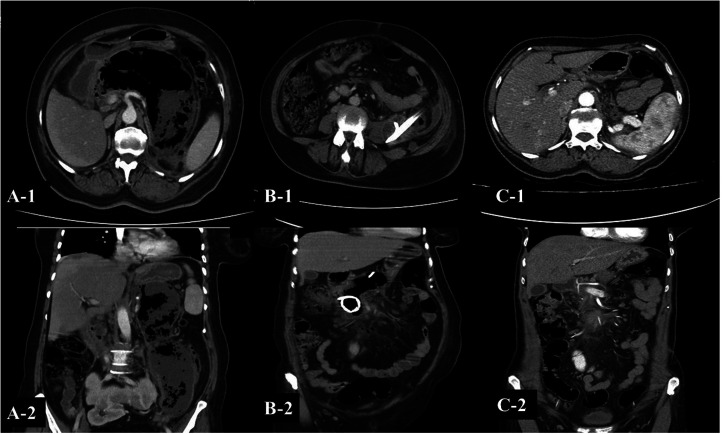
A forty-nine-year-old female patient. (A 1-2). An infected WON extending from the pancreas to the pelvis with air images inside. (B 1-2) Two percutaneous drainage catheters were inserted. (C 1-2) Complete resolution of WON was observed on follow-up CT scan 4 months after the procedure.
